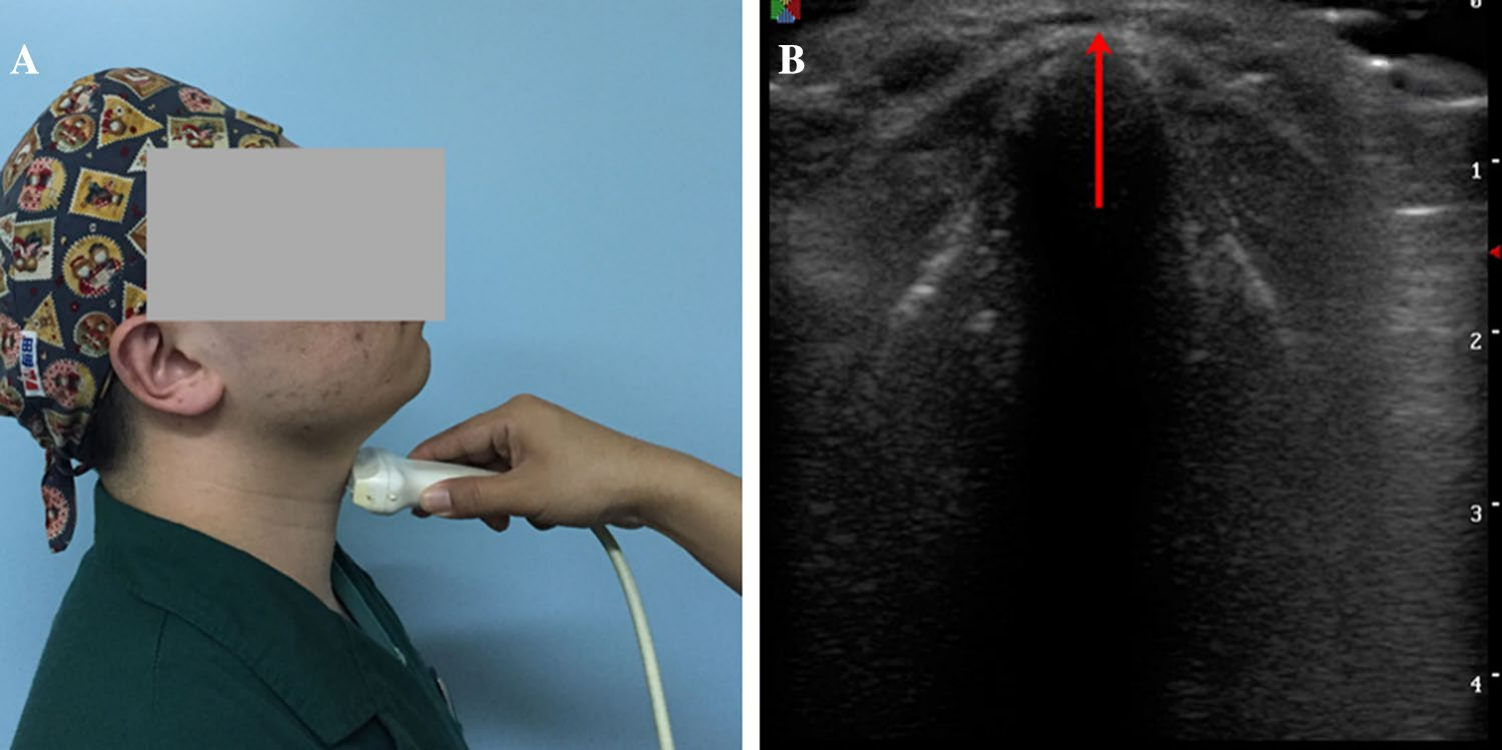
The methods used to locate the thyroid cartilage prominence. The ultrasound transducer probe was placed horizontally and moved upward to reveal the junction of the vocal cord and thyroid cartilage (indicated by the arrow)
